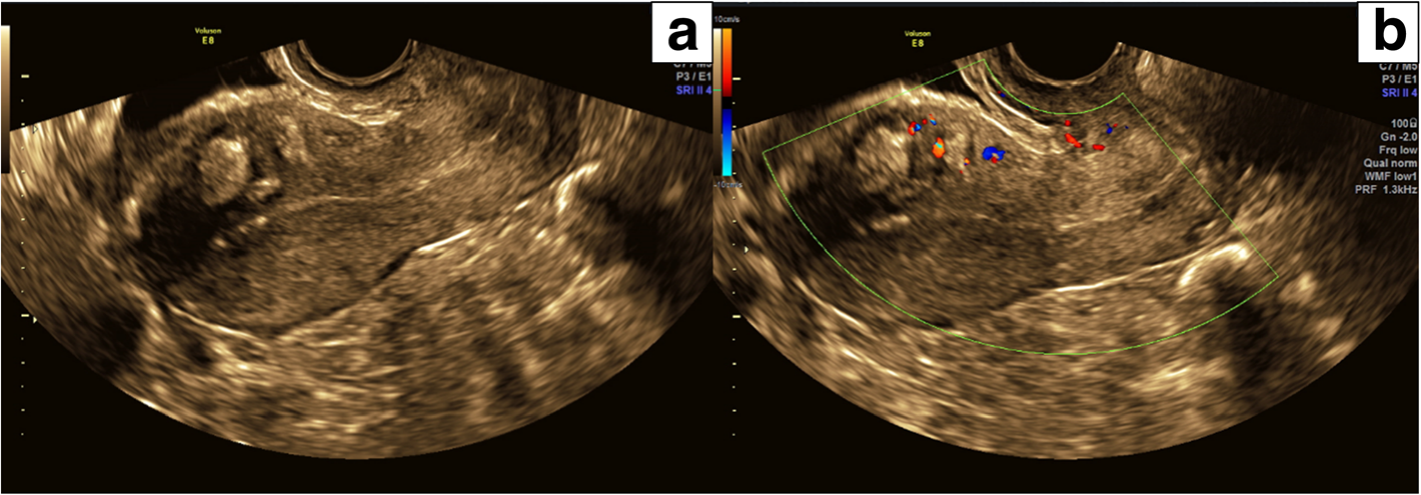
a Sagittal transvaginal pelvic ultrasound image 2 weeks post UAE demonstrating echogenic PHIL embolic material in the anterior myometrium at the site of the treated AVM b Colour Doppler overlay demonstrating markedly reduced vascularity
